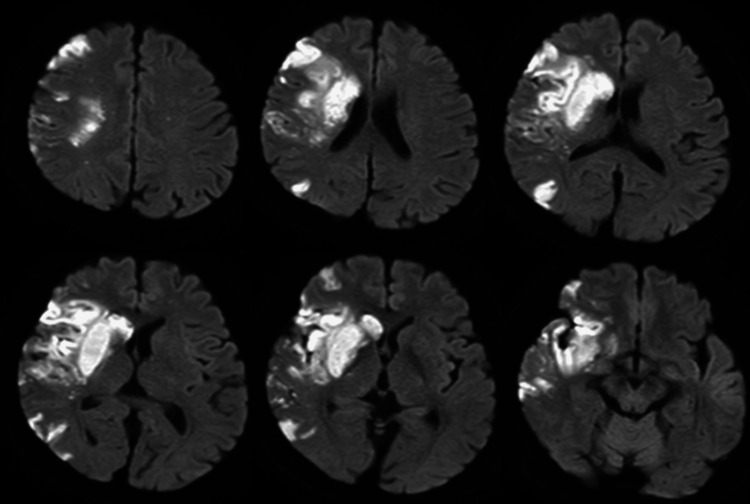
Brain images of the patient. Diffusion-weighted magnetic resonance images of the patient (day three from stroke onset).
